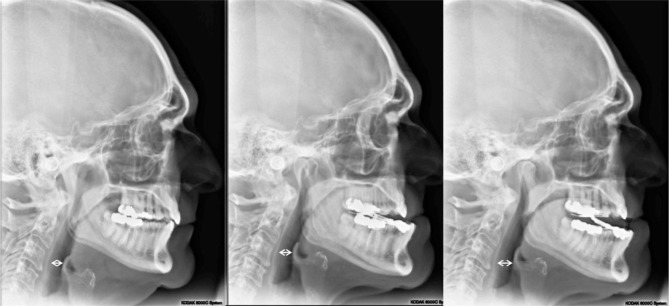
Lateral cephalometric radiographs: (Left) Pretreatment image; (Middle) minimum vertical dimension of the oral appliance (2 mm), 9 mm PAS; (Right) vertical dimension of +2 mm added to the oral appliance (4 mm total), 12 mm PAS. PAS is defined as the shortest horizontal distance between the posterior pharyngeal wall and the base of the tongue (33).
